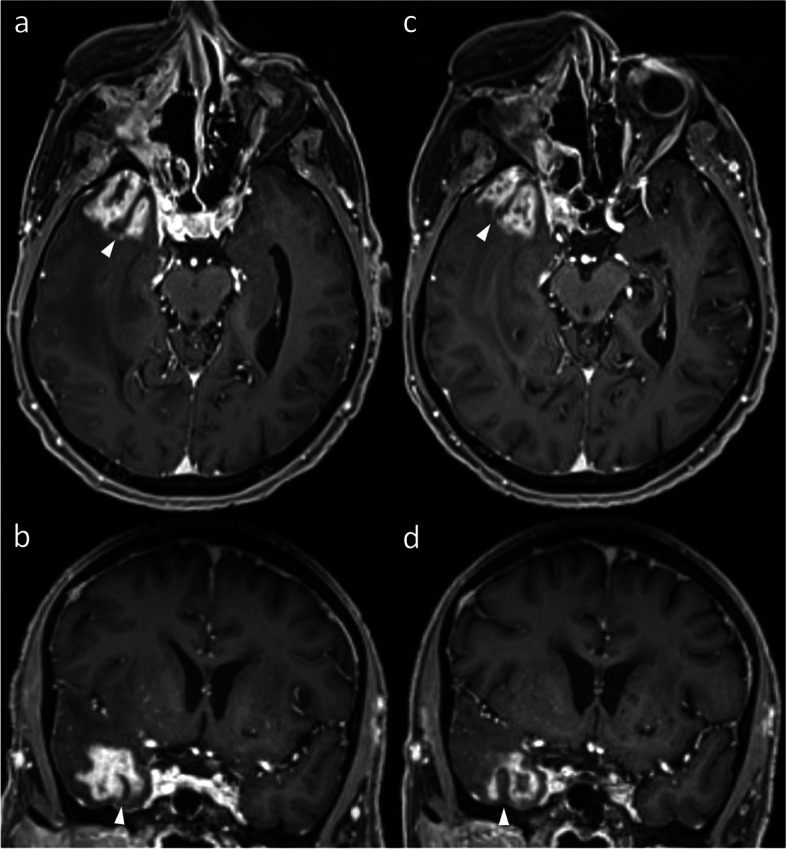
Axial (a) and coronal (b) post-contrast T1WI in a patient who was treated with a right orbital exenteration and adjuvant intensity-modulated RT (60 Gy in 30 fractions) for metastatic cutaneous squamous cell carcinoma to the medial canthus. Three years later, new enhancement developed in the right temporal lobe. While FET-PET (not shown) suggested true tumor progression, the suspicion of RN remained based on MRI, prompting a short-interval follow-up MRI (c & d). While the lesion is similar in size overall, multiplanar assessment reveals a change in the shape. The temporo-occipital sulcus (arrowheads) and adjacent cortex, which are spared, divide the overall lesion into two contiguous components: medial and lateral. While the medial component has enlarged, the lateral component has receded. The lesion continued to regress thereafter, confirming RN
